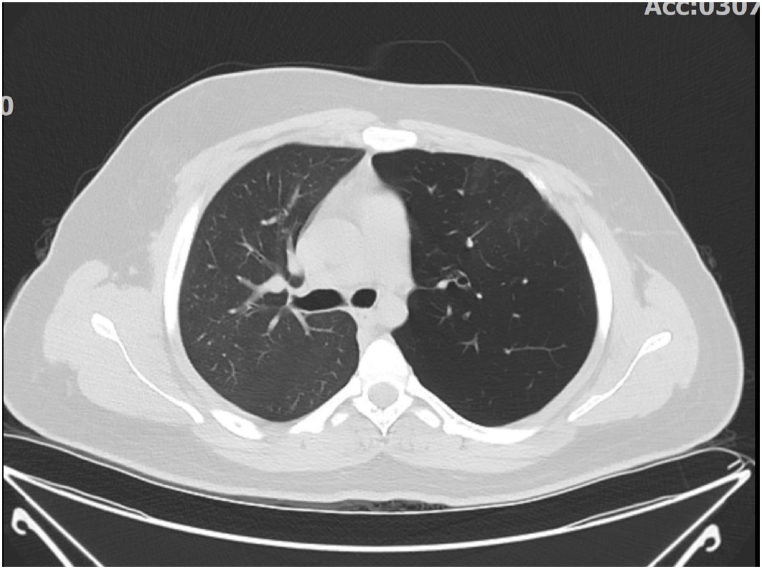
Axial HRCT. Axial view highlights expansion of the left upper lobe with rightward mediastinal displacement.
